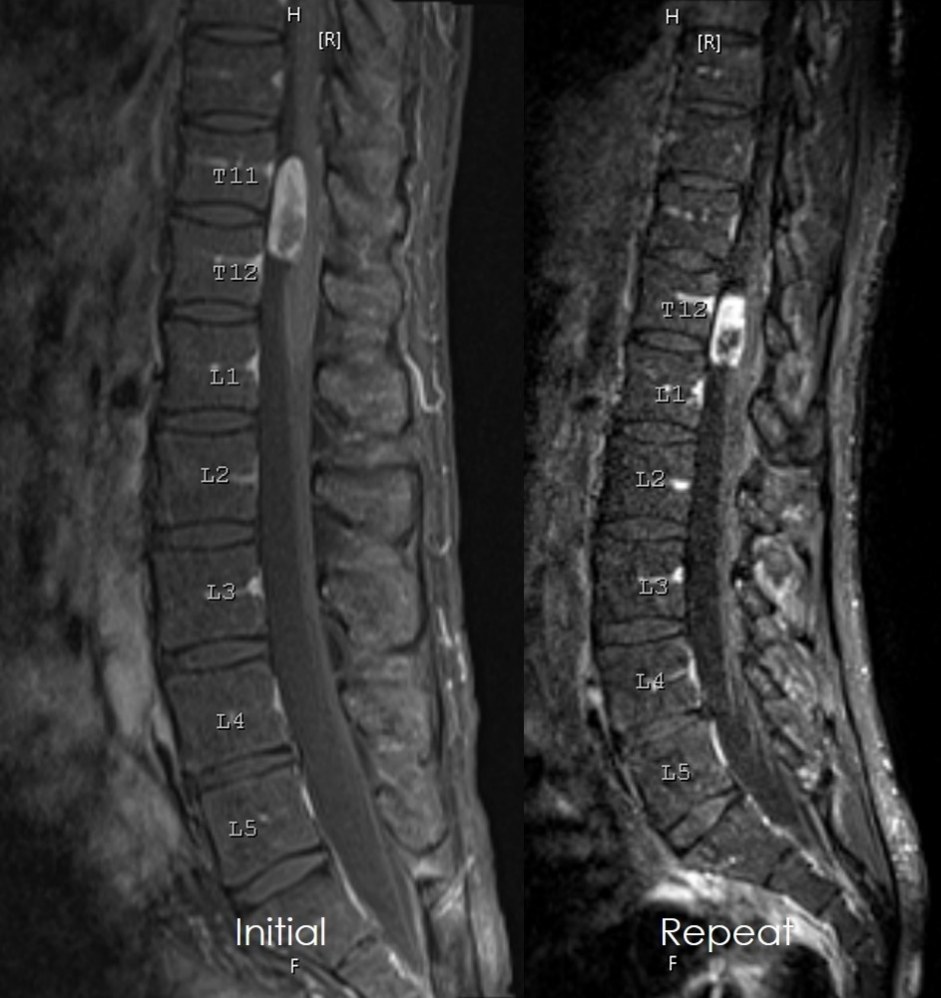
Initial and preoperative MRI imaging Initial sagittal MRI with gadolinium demonstrated an intradural extramedullary mass in the T11-T12 region. Immediate preoperative imaging obtained after new onset bladder incontinence demonstrated tumor movement caudally, down to the T12-L1 region.
